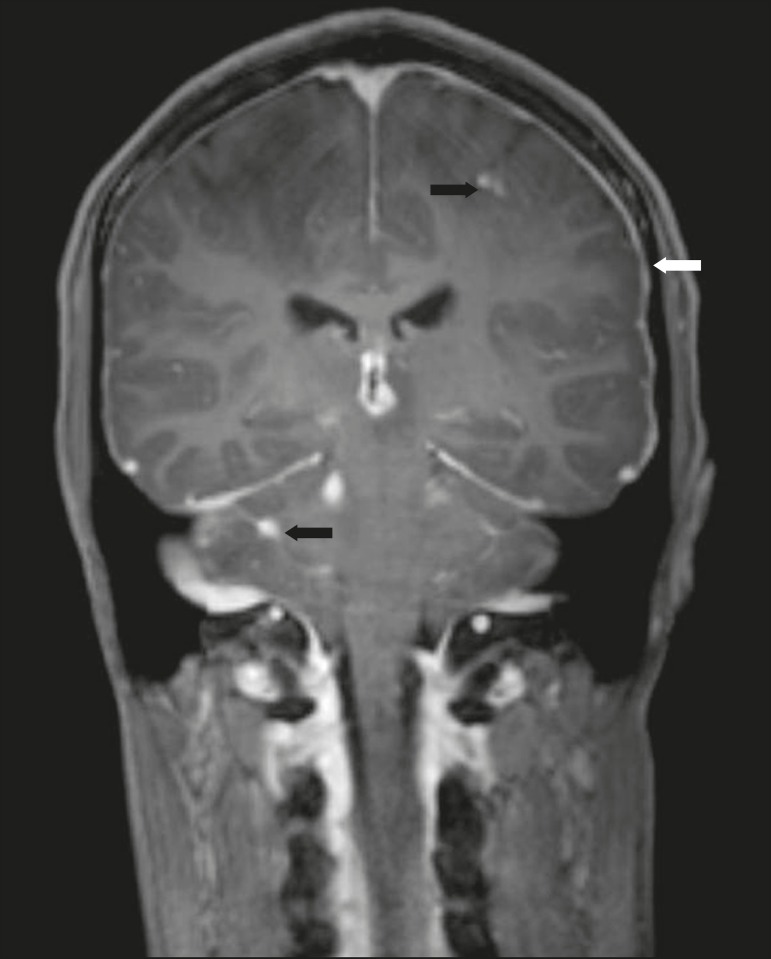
Case 2.* Contrast-enhanced coronal T1-weighted image
